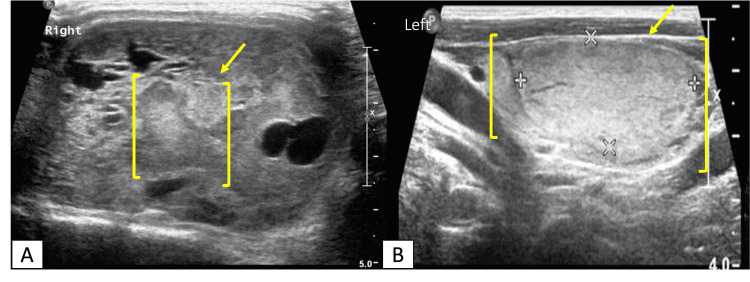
Neck ultrasound. (A) Right lobe lesion (TI-RADS 4): moderately suspicious lesion with size more than 1.5 cm. (B) Left lobe lesion (TI-RADS 3): mildly suspicious lesion with size more than 2.5 cm. TI-RADS: Thyroid Imaging Reporting and Data System
